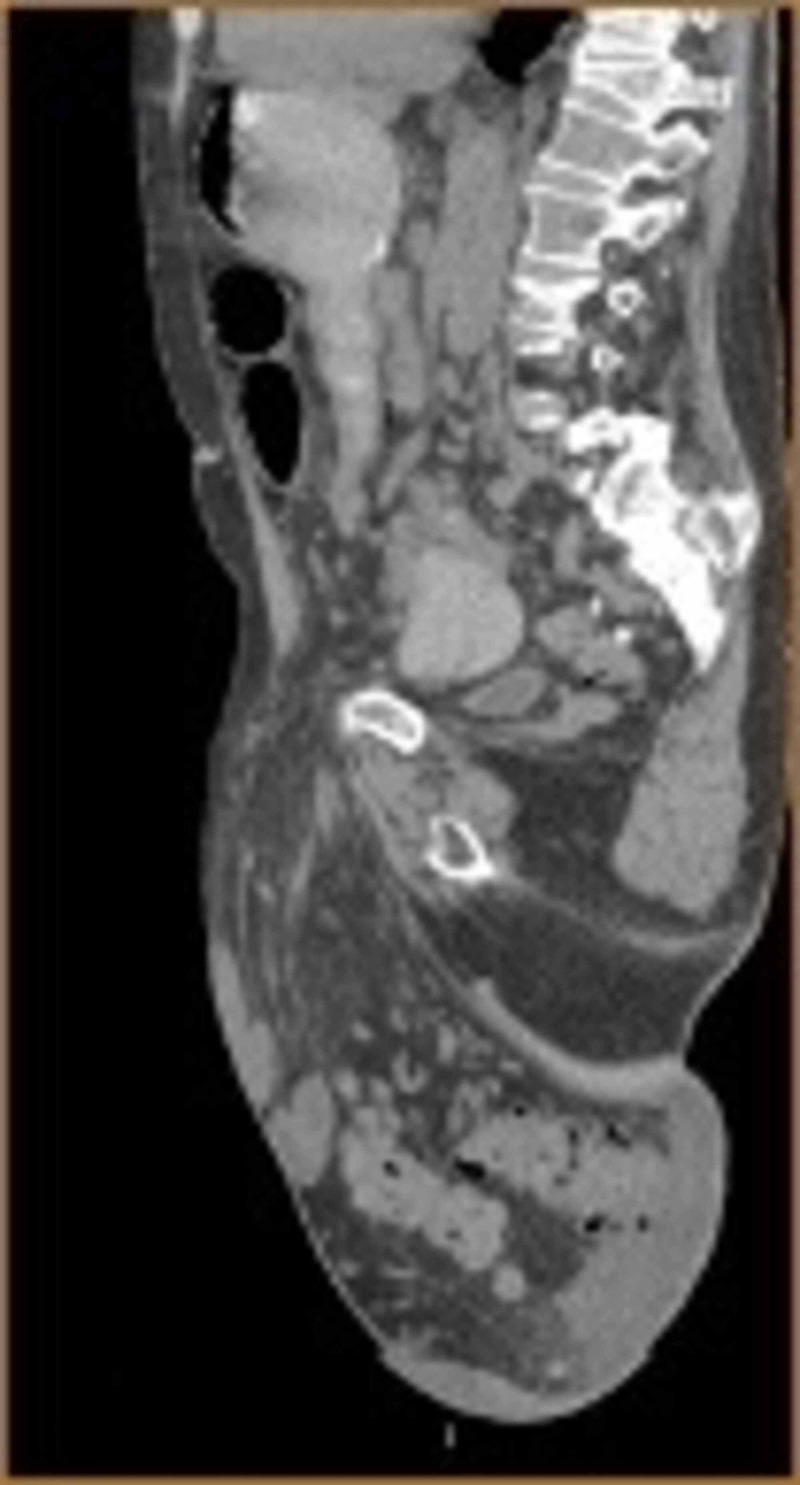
CT scan of the abdomen and pelvis (sagittal view) demonstrating massive scrotal contents
